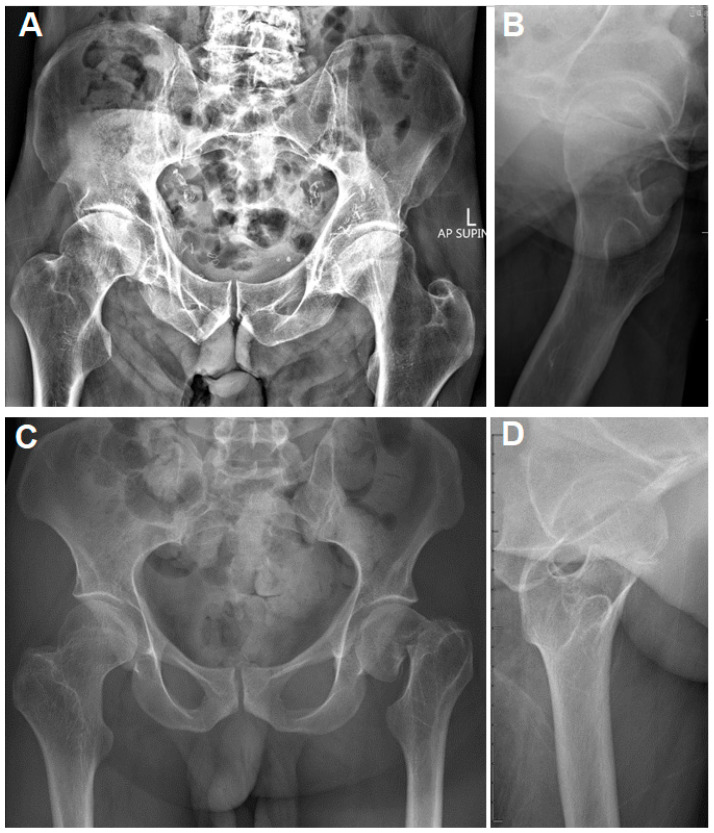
(A,B): Plain pelvis radiographs of a 71-year-old male with CP and a right valgus-impacted, ventrally tilted femoral neck fracture. The contralateral hip indicates an elevated femoral neck–shaft angle. (C,D): Plain pelvis radiographs of a 33-year-old male with CP and a left displaced femoral neck fracture. The contralateral femoral neck–shaft angle is increased as well.
